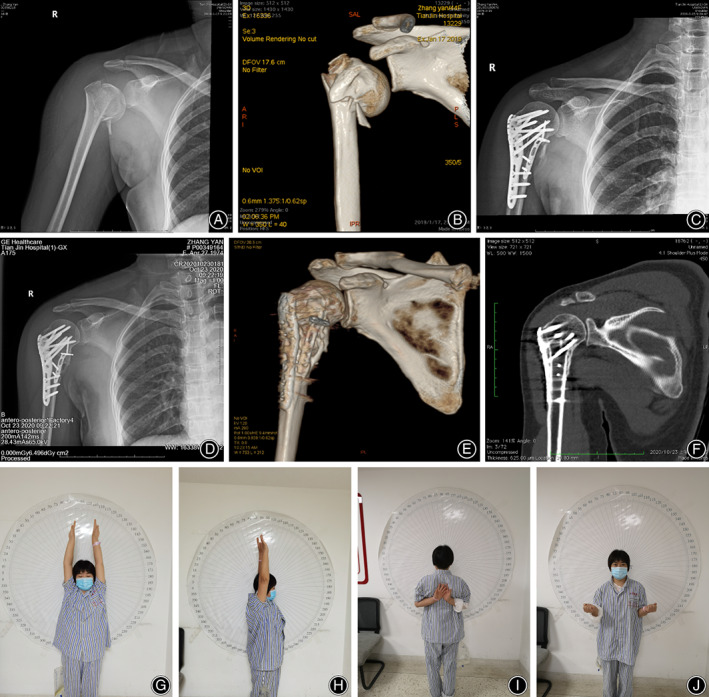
The patient was a 44‐year‐old female with a proximal humerus fracture (Neer classification: three‐part fracture). (A, B) Frontal X‐ray images and computed tomography (CT) images of the right shoulder joint after a fall on the right shoulder, showing a comminuted fracture of the proximal end of the right humerus and a comminuted calcar. (C) X‐ray image taken immediately after the operation, showing that the proximal humerus is well‐reduced and the neck‐shaft angle is restored. (D–F) X‐ray images in the anteroposterior (AP) view and the CT image showed: plate position was satisfactory and bone union was successful. (G–J) Show that 1 year after the operation, the shoulder joint could be flexed and lifted up to 180°, the internal rotation reached the T4 level, the lateral external rotation was 30°, and the functional recovery was satisfactory.
